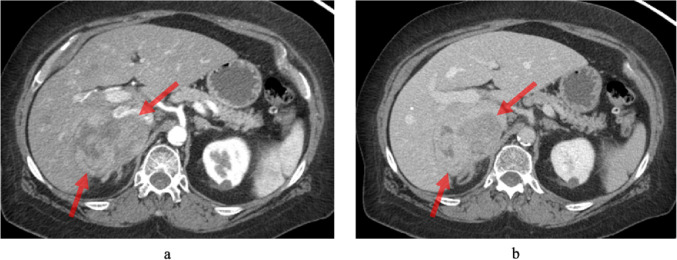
Axial contrast-enhanced CT images one level below that of Fig. 5 in arterial (a) and portal venous phases (b) demonstrate a large contiguous extravascular tumor component with similar enhancement characteristics (red arrows)
